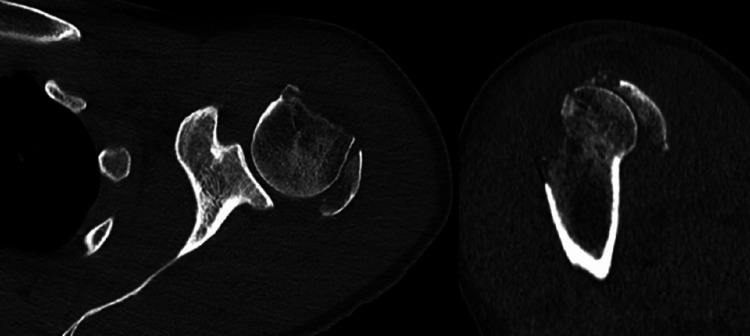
CT scan axial and sagittal images, obtained on presentation to the upper limb fracture clinic These demonstrate significant displacement of the greater tuberosity fragment in a posterior and superior direction, relevant to the humeral head. Healing in such a position, the patient would experience a mechanical block to external rotation and significant weakness, secondary to mechanical disadvantage of the rotator cuff tendons. CT: Computerized tomography
